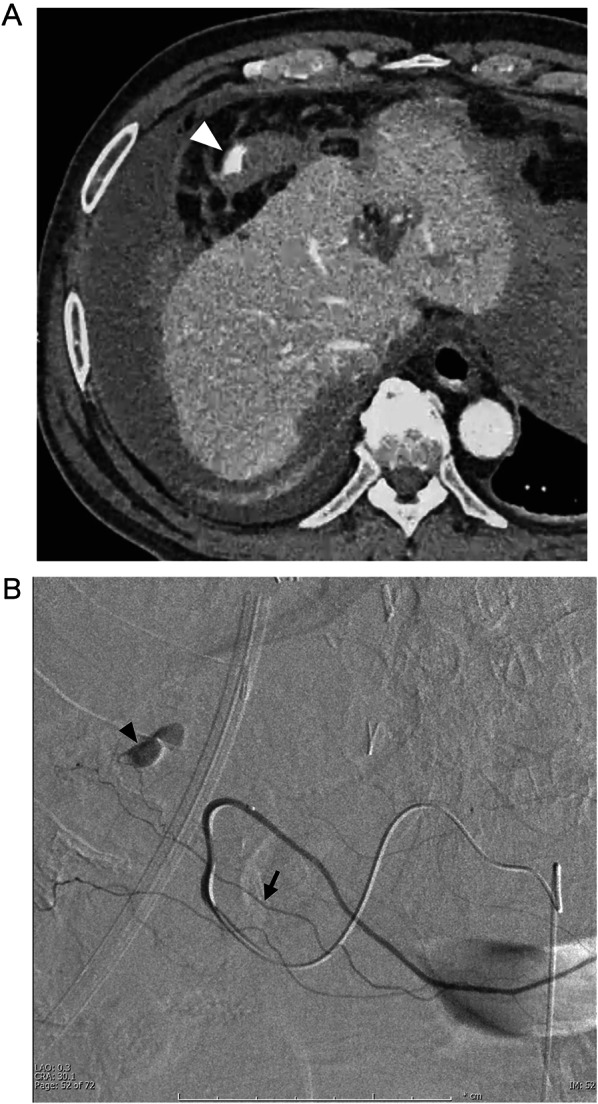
Radiological imaging of the postoperative day 1. A Contrast-enhanced CT. The arrowhead indicates extravasation from the greater omentum. B Emergency interventional radiology findings. The arrowhead indicates extravasation from the branch (arrow) of the right gastroepiploic artery. The branch was embolized peripherally, and the right gastroepiploic artery was preserved
